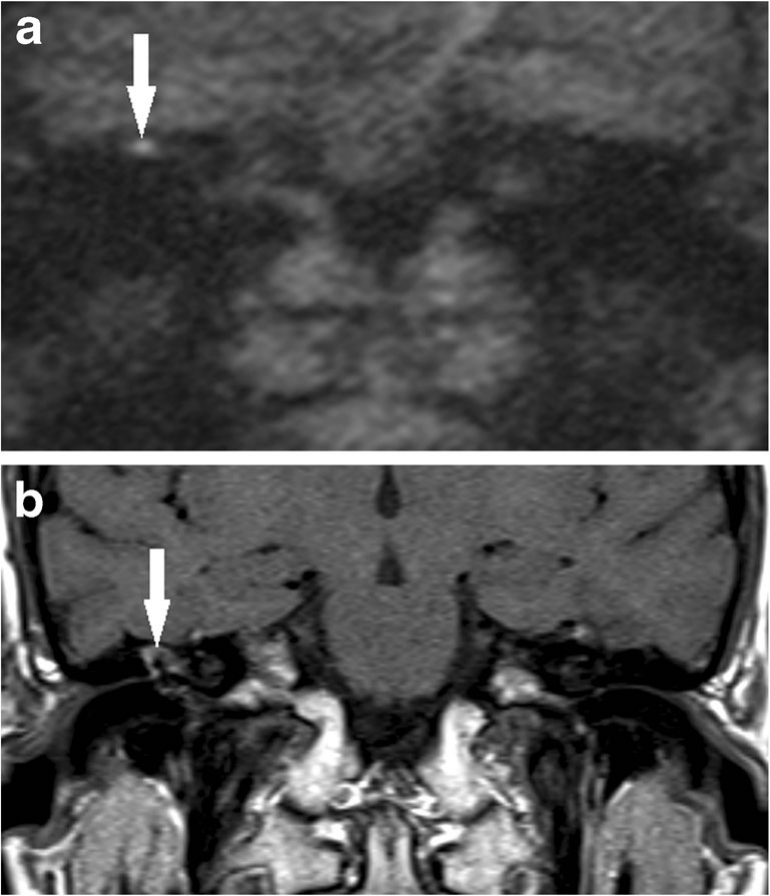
False positive high DWI signal for cholesteatoma. a The coronal b1000 image shows high signal at the epitympanum, which raised suspicion for residual cholesteatoma. However, b the corresponding non-contrast enhanced coronal T1 weighted image showed high signal (*white arrow*) and the ADC map intermediate signal and value, both *not supporting* the diagnosis of cholesteatoma. Surgery confirmed non-specific inflammation with no cholesteatoma
